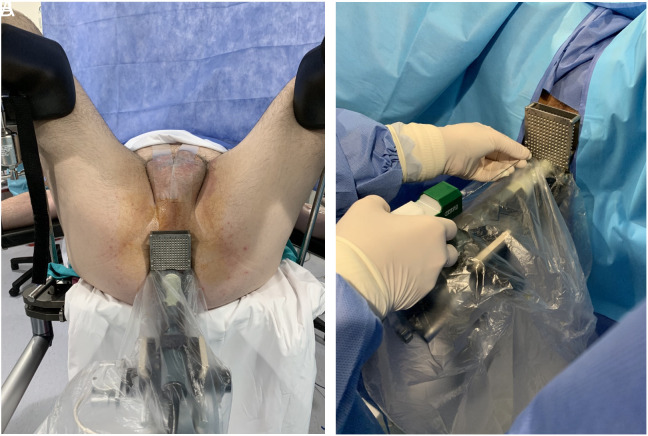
A, B. (A) The patient is positioned in a lithotomy forced position, with the scrotum elevated using adhesive tape to maximize exposure of the perineum. (B) A mechanical arm is used, onto which the ultrasound transducer is mounted, and a metal grid is attached adjacent to the perineum.
